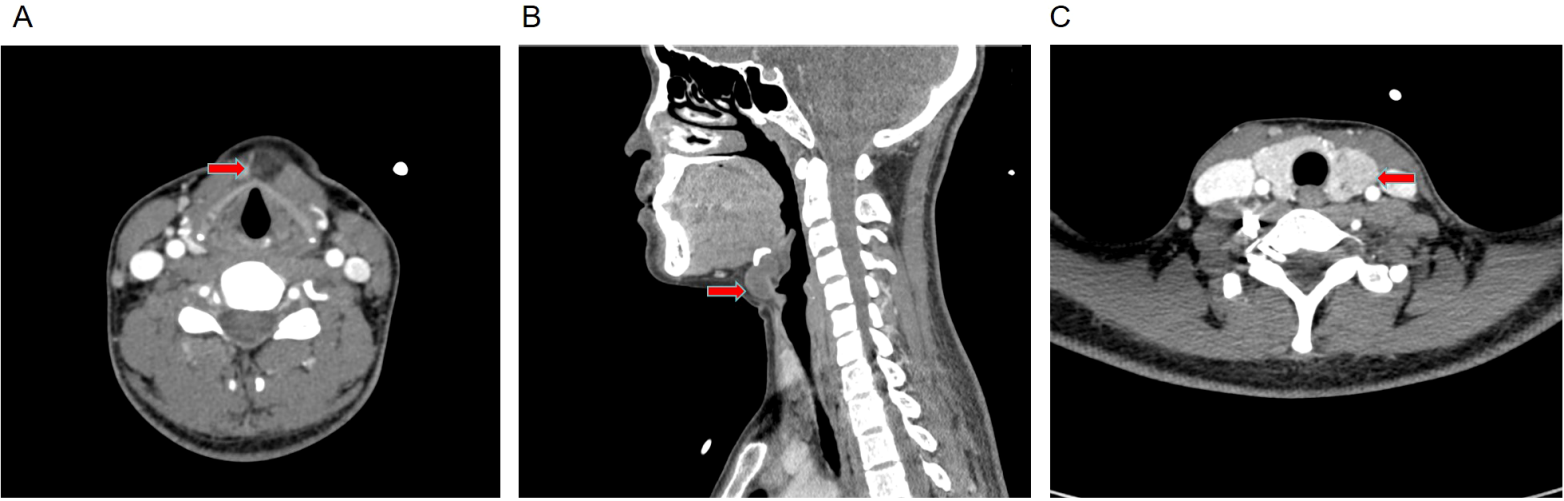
Computed tomography findings of the cervical mass. (A) Low-density cystic cavity is present in the hyoid cartilage-thyroid cartilage space, measuring 12×18×22 mm, with a CT value of 25 HU. (B) The boundary is clear and there is no enhancement on enhanced scanning. (C) An irregular low-density mass of 20×20×30mm in size was found in the left lobe of the thyroid gland. The boundary was unclear. The enhanced scan showed significant uneven enhancement, and the CT value was still lower than that of the normal thyroid gland.
